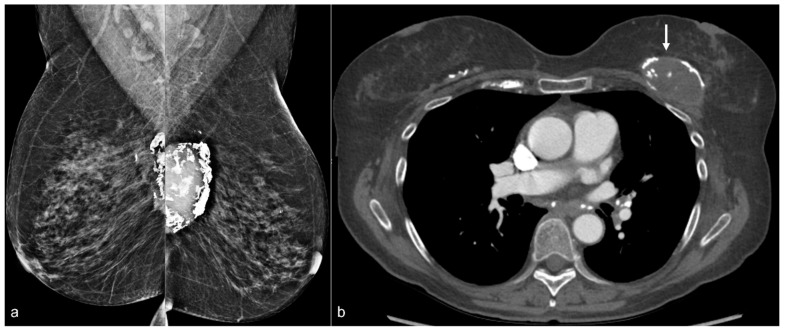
A 70-year-old patient’s mammogram (a) demonstrates bilateral calcified implant capsules with a dense mass in the left prepectoral location on the MLO view. CT (b) confirms a left breast seroma (arrow) with fibrous capsular calcifications.
